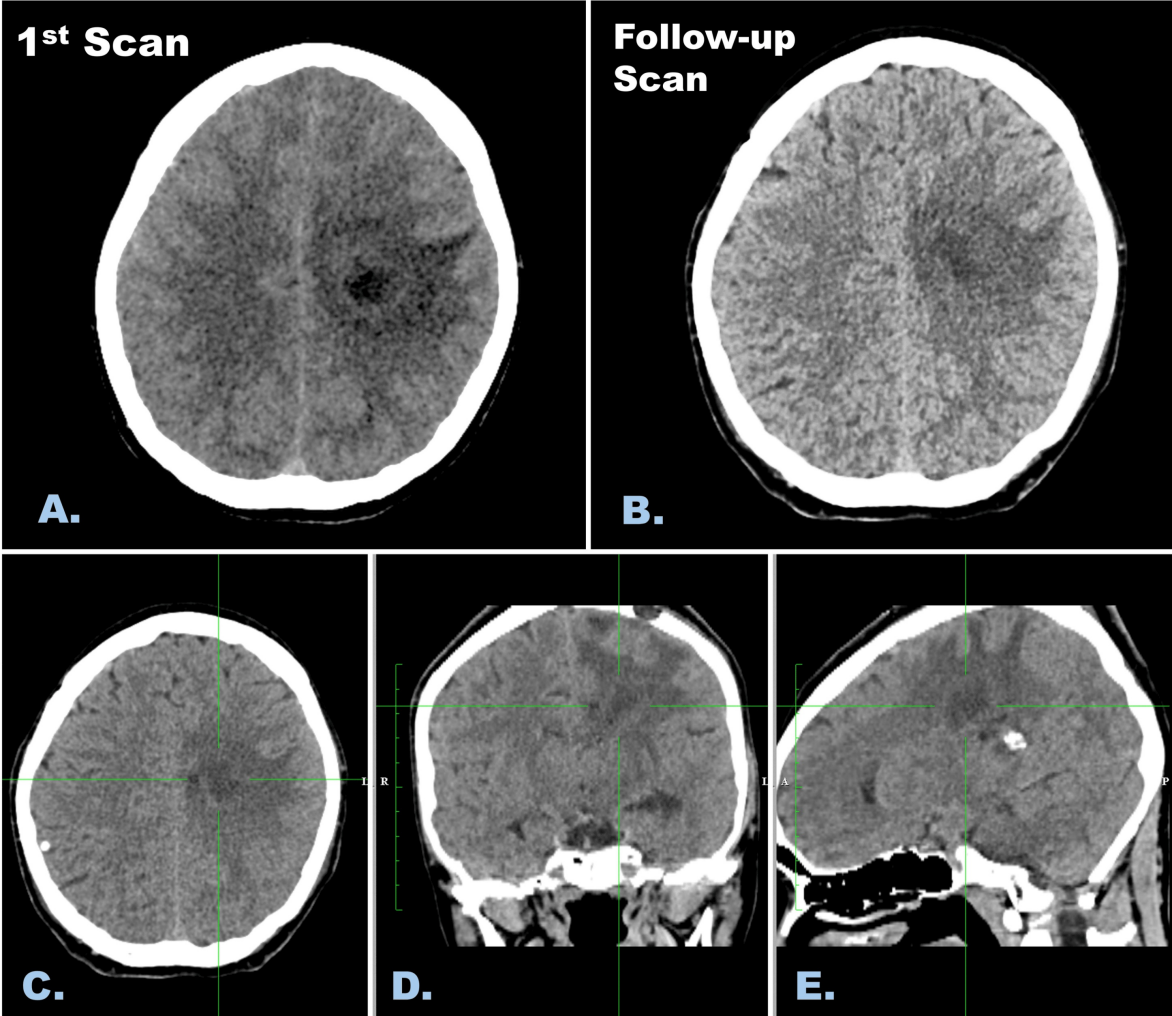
Initial non-enhanced CT of the brain showing lesion in the left centrum semiovale and corona radiata, accompanied by perilesional edema (A). A follow-up non-enhanced CT of the brain after one week showing a significant reduction in the lesion size and perilesional edema (B). Axial (C), coronal (D), and sagittal (E) multiplanar reconstructed images of the follow-up scan. CT: computed tomography
